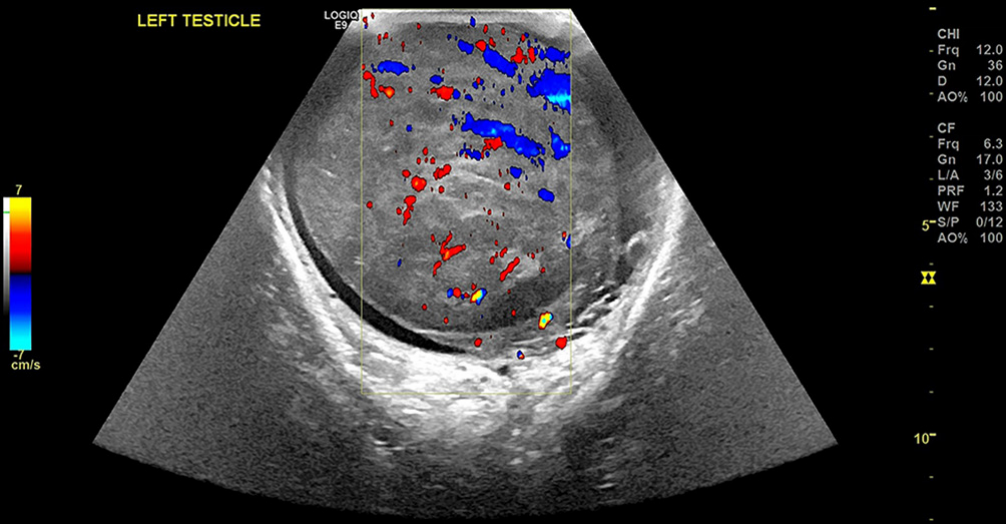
Colour doppler with the spectral gate over the left testicle demonstrating disorganised hypervascularity.
